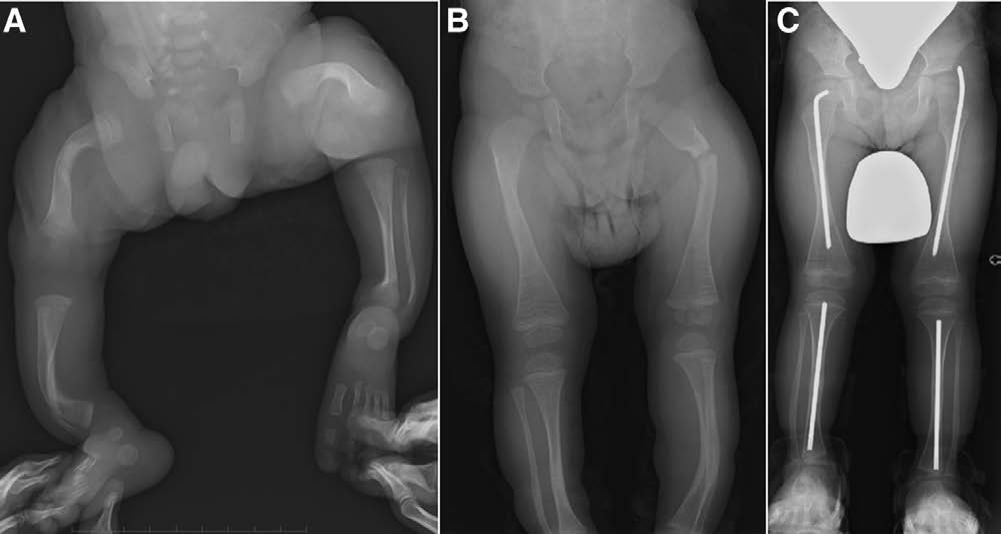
Serial radiographs of an 8-year-old boy with osteogenesis imperfecta. A neonatal anteroposterior radiograph of the lower extremities demonstrating bowing of the long bones of the lower extremities (A) The patient’s birth height was 46.0 cm (−1.3 SD). An anteroposterior radiograph of the lower extremities at the age of 2.1 years showing a left femoral fracture that required corrective osteotomy with intramedullary fixation (B). His height at the first surgery was 78.5 cm (−3.0 SD). An anteroposterior radiograph of the lower extremities at the age of 8.3 years revealing bilateral intramedullary rodding for the femora and tibiae, and the patient was able to walk independently (C).
